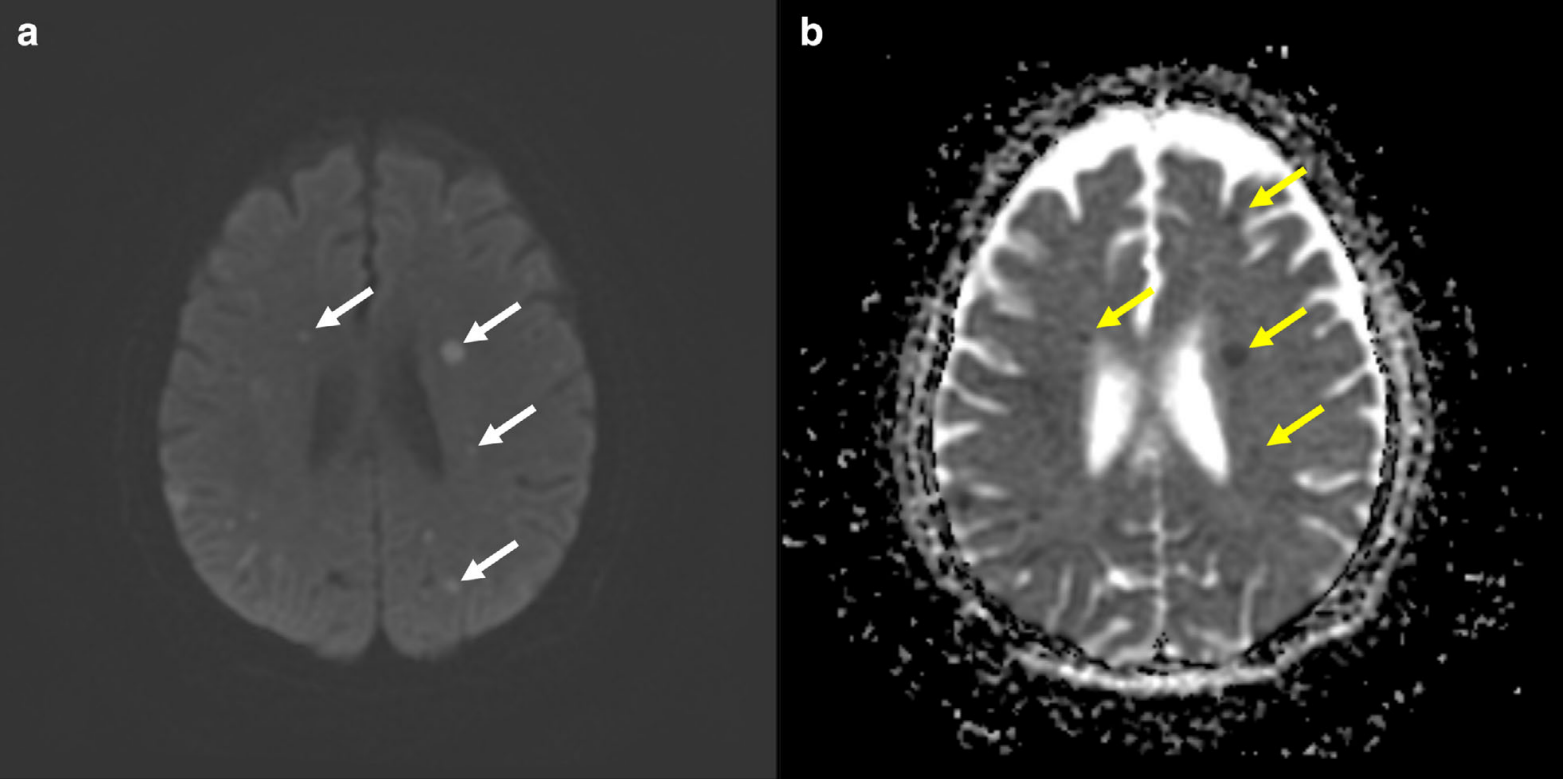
Sequential diffusion‐weighted imaging (a) and the apparent diffusion coefficient (b) of the brain magnetic resonance imaging showed an increased multifocal signal because of diffusion restriction and a corresponding decrease in signal intensity, respectively. The result is compatible with ischemic stroke‐related cytotoxic edema.
